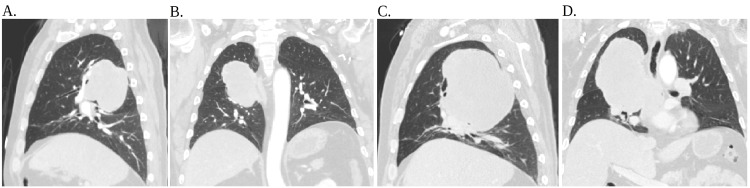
CT scan of the chest, abdomen, and pelvis (A) CT of the chest with contrast sagittal view shows a large right upper lobe/lower lobe pulmonary mass measuring 8×6×7 cm concerning for primary lung neoplasm. (B) CT of the chest with contrast transverse view shows a large right upper lobe/lower lobe pulmonary mass measuring 8×6×7 cm concerning for primary lung neoplasm. (C) CT of the chest with contrast sagittal view shows a pre-existing mass at the posterior right upper and mid-lung zone with contiguous posterior mediastinal extension which increased in size at a visit approximately one year later, now measuring 11×10×13 cm. (D) CT of the chest with contrast transverse view shows a pre-existing mass at the posterior right upper and mid-lung zone with contiguous posterior mediastinal extension which increased in size at a visit approximately one year later, now measuring 11×10×13 cm. CT: computed tomography
